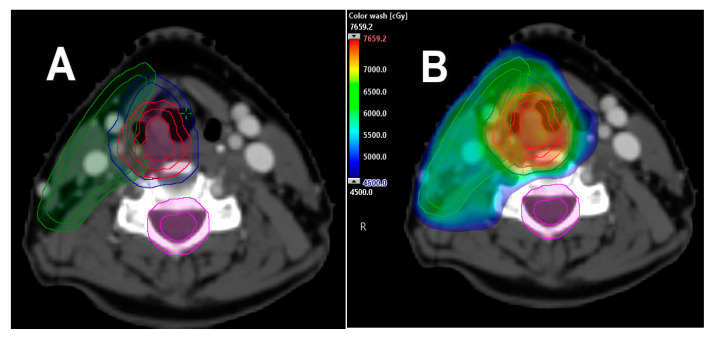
Exemplification of Unilateral neck irradiation case. Unilateral neck irradiation treatment plan for T1N0 squamous cell carcinoma of the right supraglottic larynx, after SPECT/CT with peritumoral 99mTc-nanocolloid injection for lymph drainage mapping. Prescription dose 70 Gy in 35 fractions to high risk PTV. (A) Delineation of GTV, high risk CTV/PTV (red), intermediate (blue), and low (green) utilizing 5 mm uniform expansion to render PTV’s. (B) VMAT treatment planning technique with partial arcs delivered via simultaneously integrated boost method. Dose color wash distribution.
